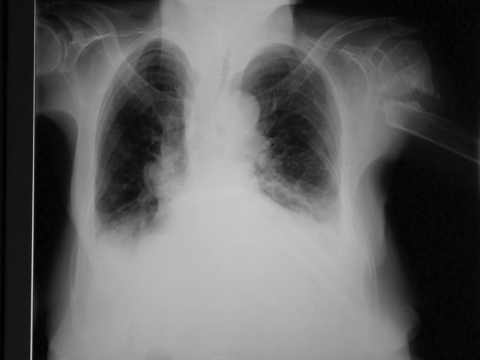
Chest radiograph at first admission.
